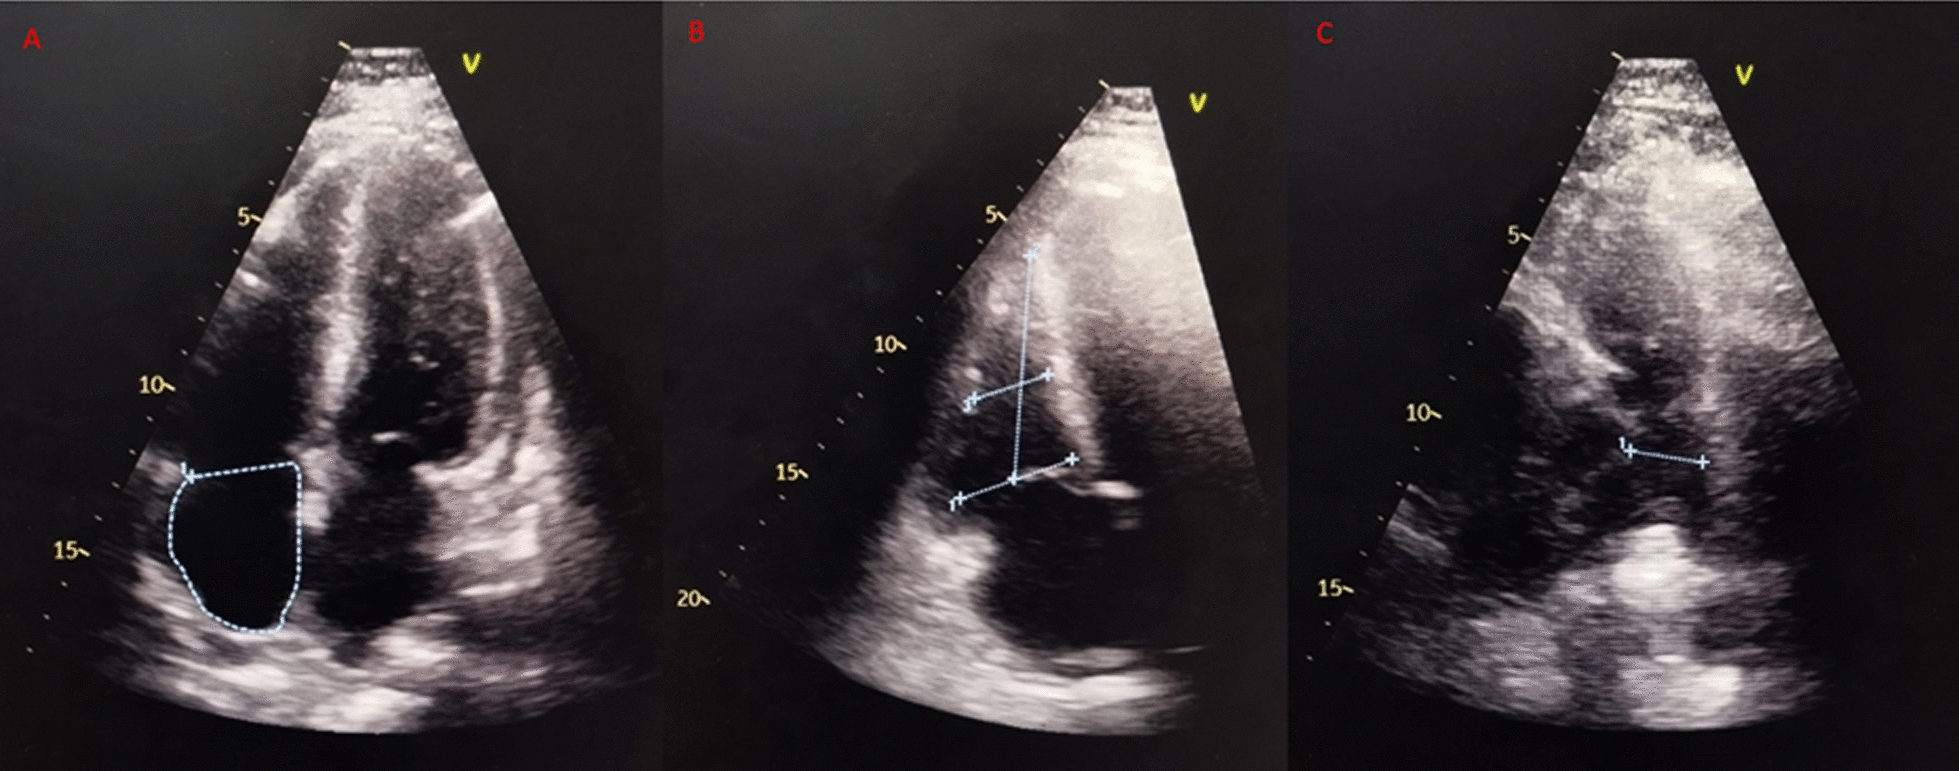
The echocardiography showed (A) a right atrium area of 12.5 cm^2^, (B) a right ventricle basal diameter of 37 mm, and (C) a main pulmonary artery diameter of 19 mm
